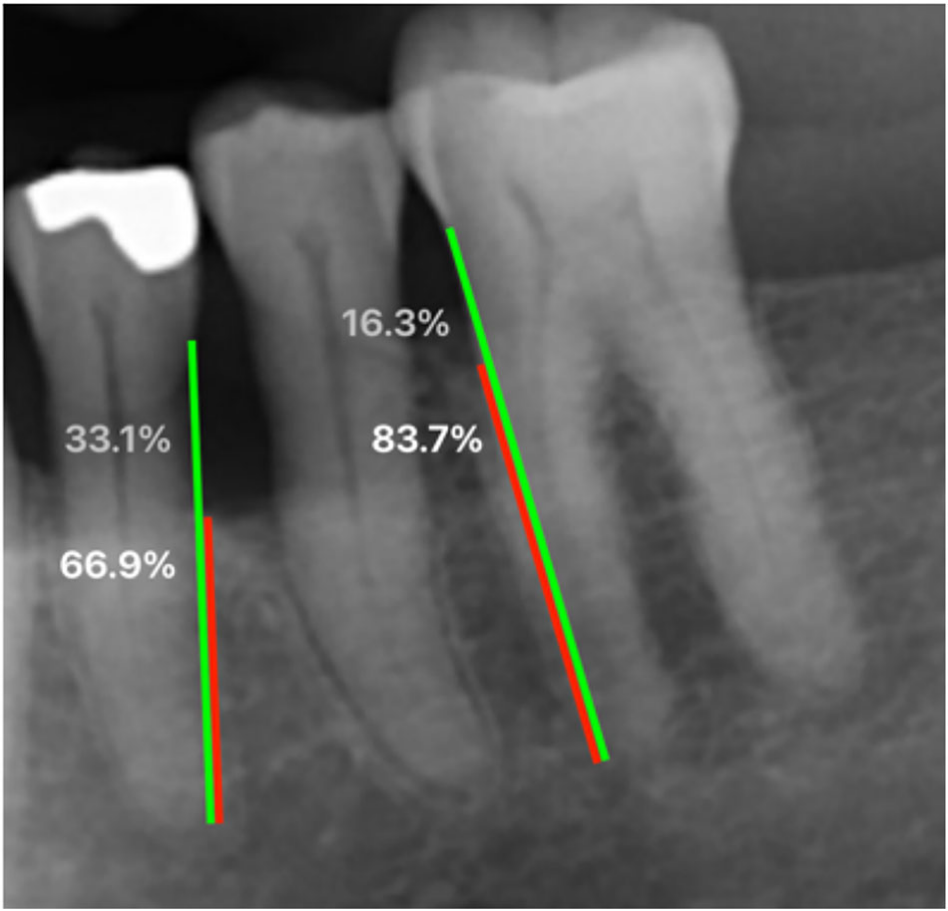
Radiographic measurements. Radiographic assessments were performed by measuring the distance from the marginal alveolar bone to the tooth apex (red line) and from the cementoenamel junction to the tooth apex (green line). The proportions of remaining bone and bone loss are depicted in white and gray, respectively. Bone loss exceeding 33% (as presented at the first premolar in this figure) were used as a threshold.
